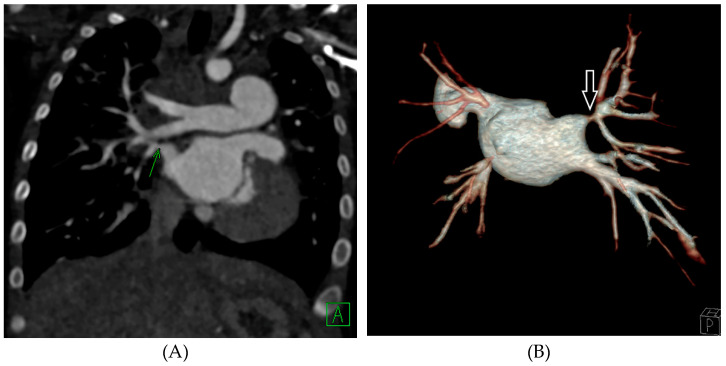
(A): Coronal slice of a CT angiogram demonstrates right upper pulmonary vein stenosis (green arrow). (B): Posterior view of the three-dimensional reconstruction of the CT angiogram of the same patient also demonstrates right upper pulmonary vein stenosis (white arrow), in addition to severe stenosis of the left lower pulmonary vein and atresia of the left upper pulmonary vein.
